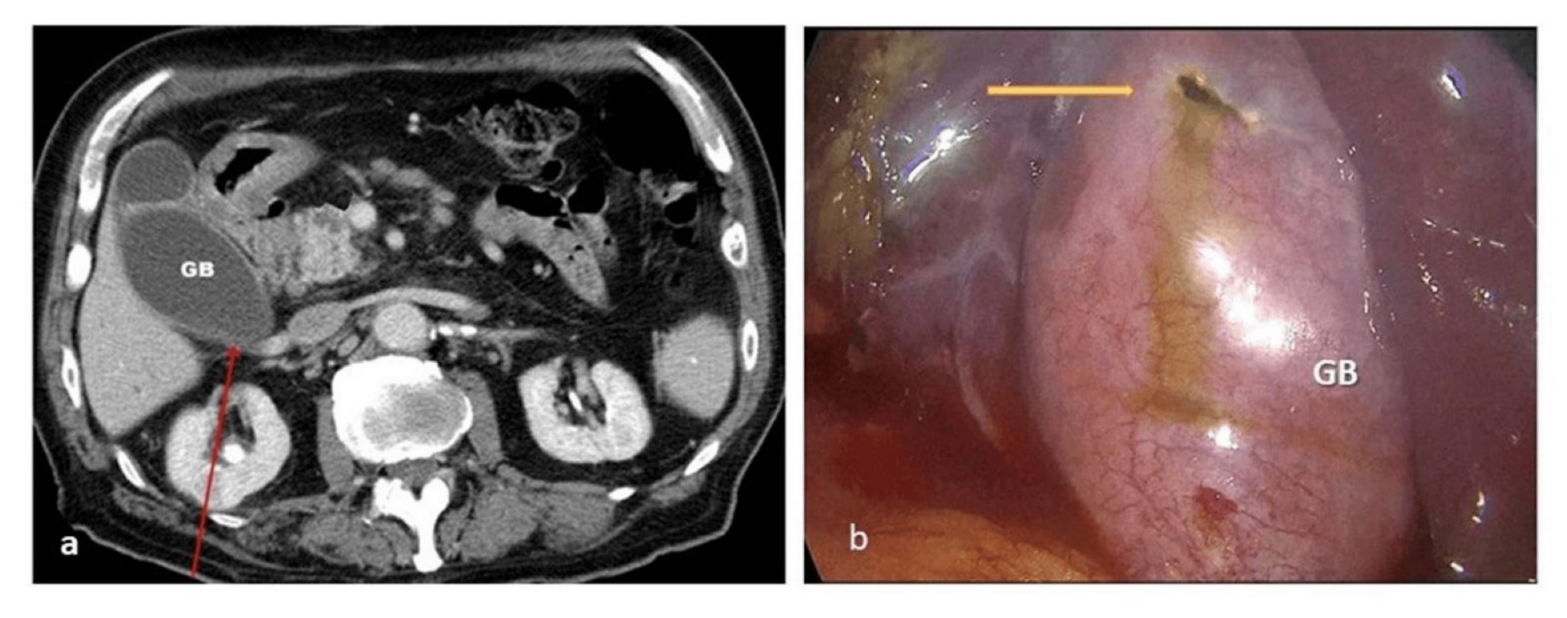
Relationship between the right kidney and the gall bladder (GB), and an intra-operative finding of the diagnostic laparoscopy a) Axial computed tomography (CT) image of the abdomen showing the relationship between the right kidney and the GB (red arrow). b) Intra-operative finding of the GB perforation site with bile leak (yellow arrow).
